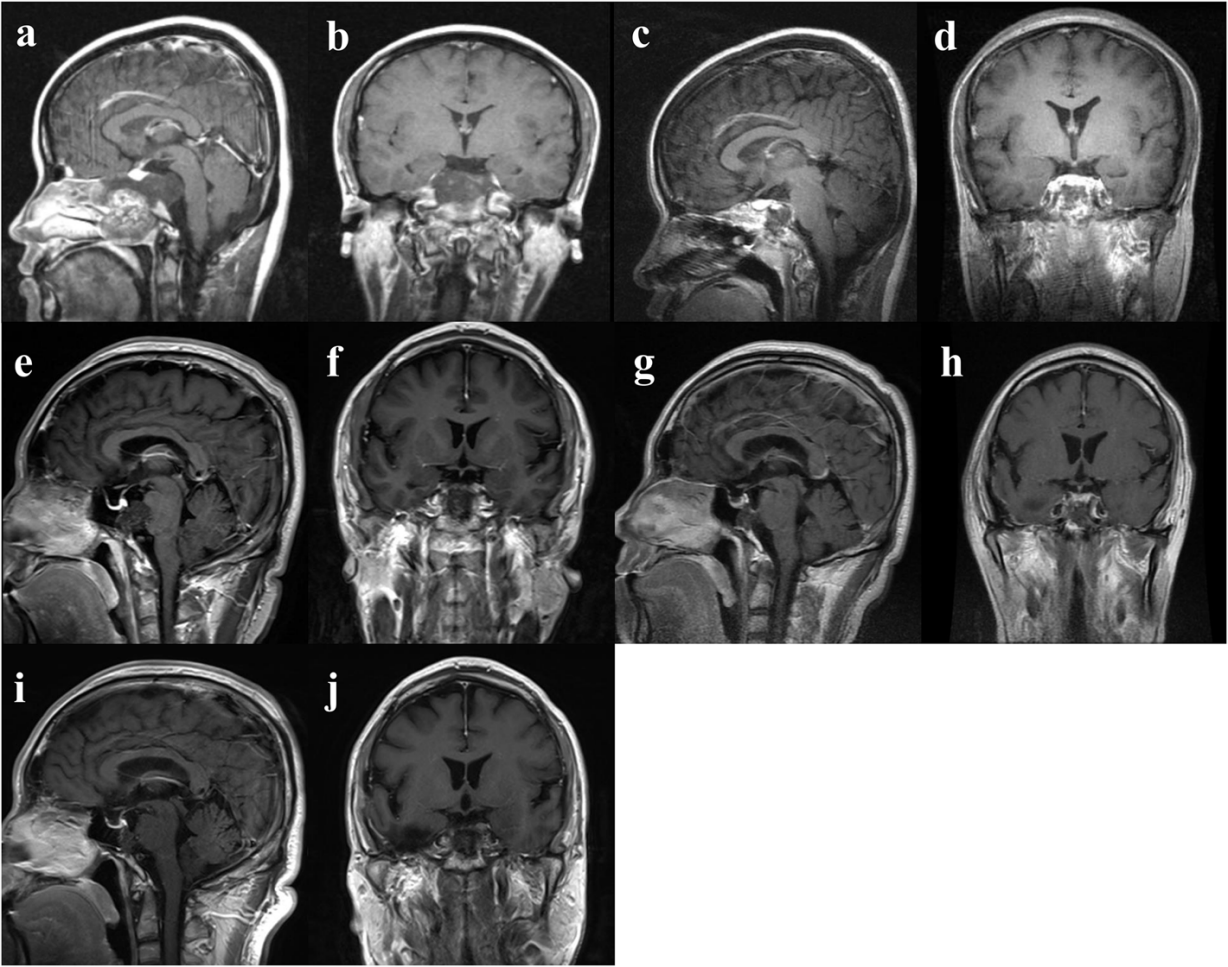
Representative preoperative and postoperative MR images showing the definition of total and non-total resection, as well as the value of combined treatment. a-d preoperative and postoperative images of one case who received total resection and the patient got no recurrence during the follow up. e-h preoperative and postoperative images of another case who received non-total resection and following adjuvant radiotherapy. i-j After surgery and postoperative adjuvant radiotherapy, the case shown in e-h had no sign of recurrence on the 70 months follow up images
